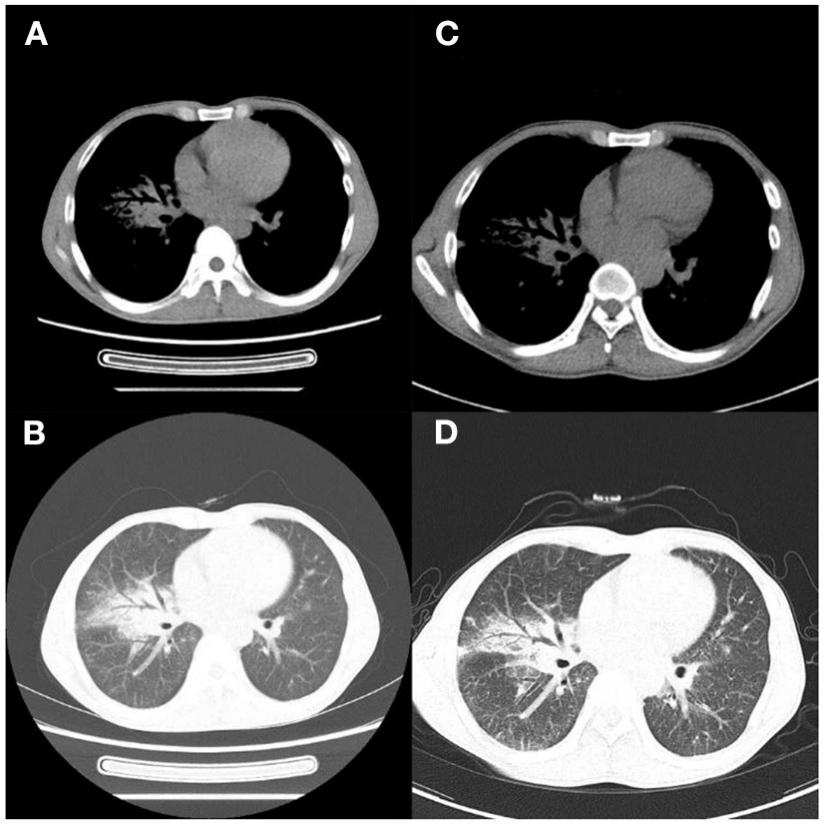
Case 1. (A,B) Chest CT 4 months later showed improved lung lesions; (C,D) and scar formation in the right middle lobe bronchus 8 months later.
